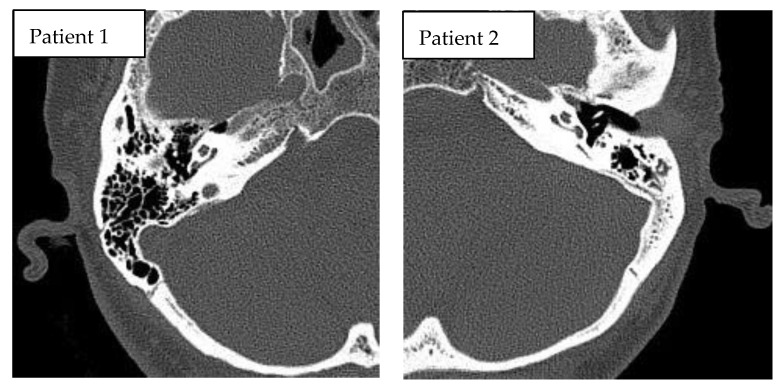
Preoperative CT of temporal bones of the patients, both cases with normal anatomy of the temporal bone.
